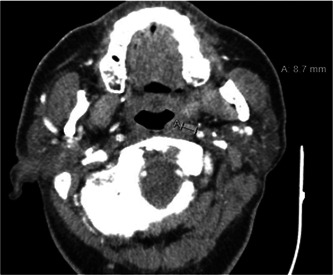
Preoperative computed tomography neck demonstrating left retropharyngeal lymph node (denoted by the letter “A”), positive for squamous cell carcinoma on surgical pathology.
